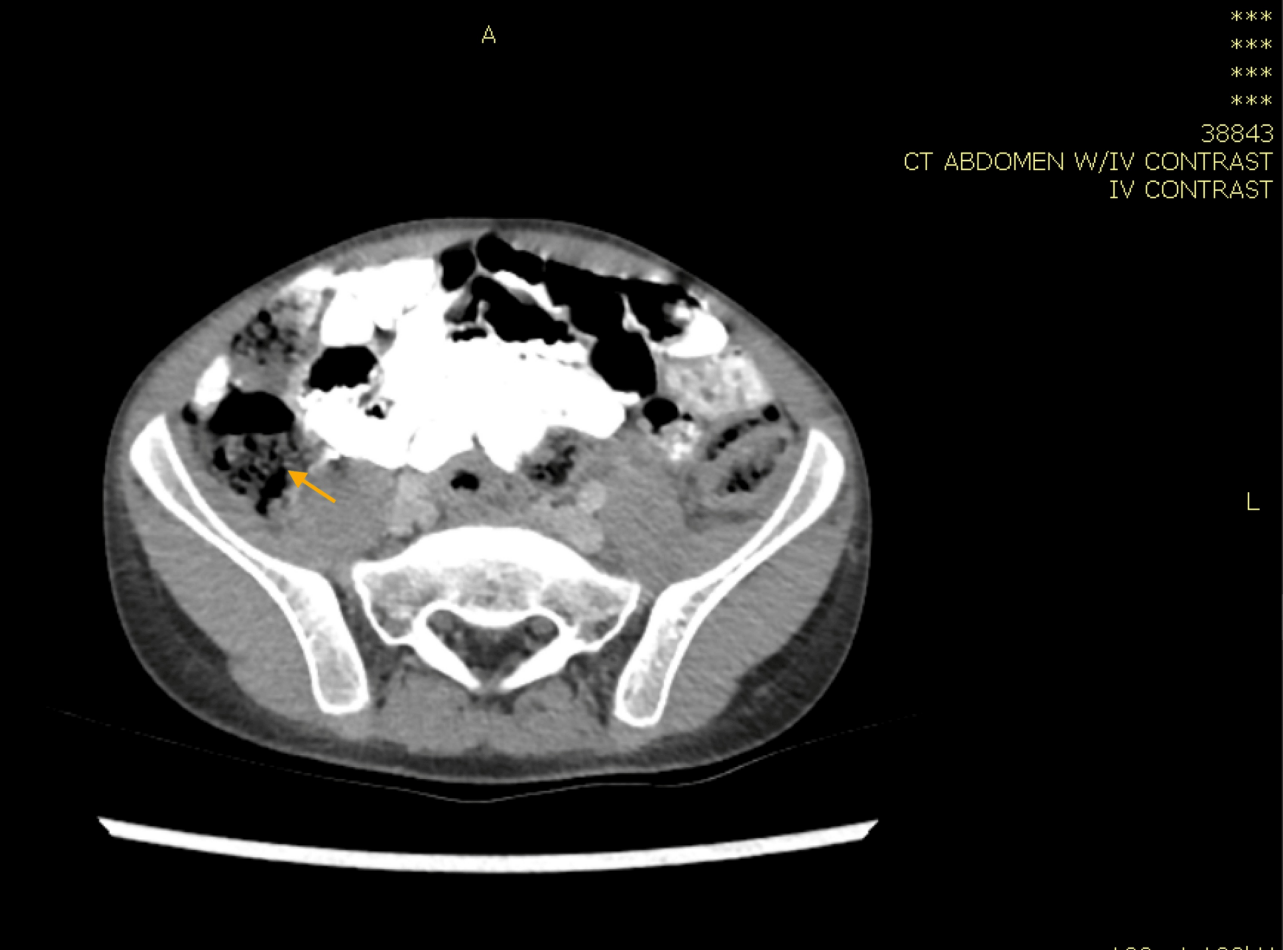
Computed tomography scan of the abdomen with IV contrast revealing no evident bowel wall thickening (orange arrow) and no further hepatic lesions. The patient was put on regular radiological and clinical follow up every three months with no features of relapse.
